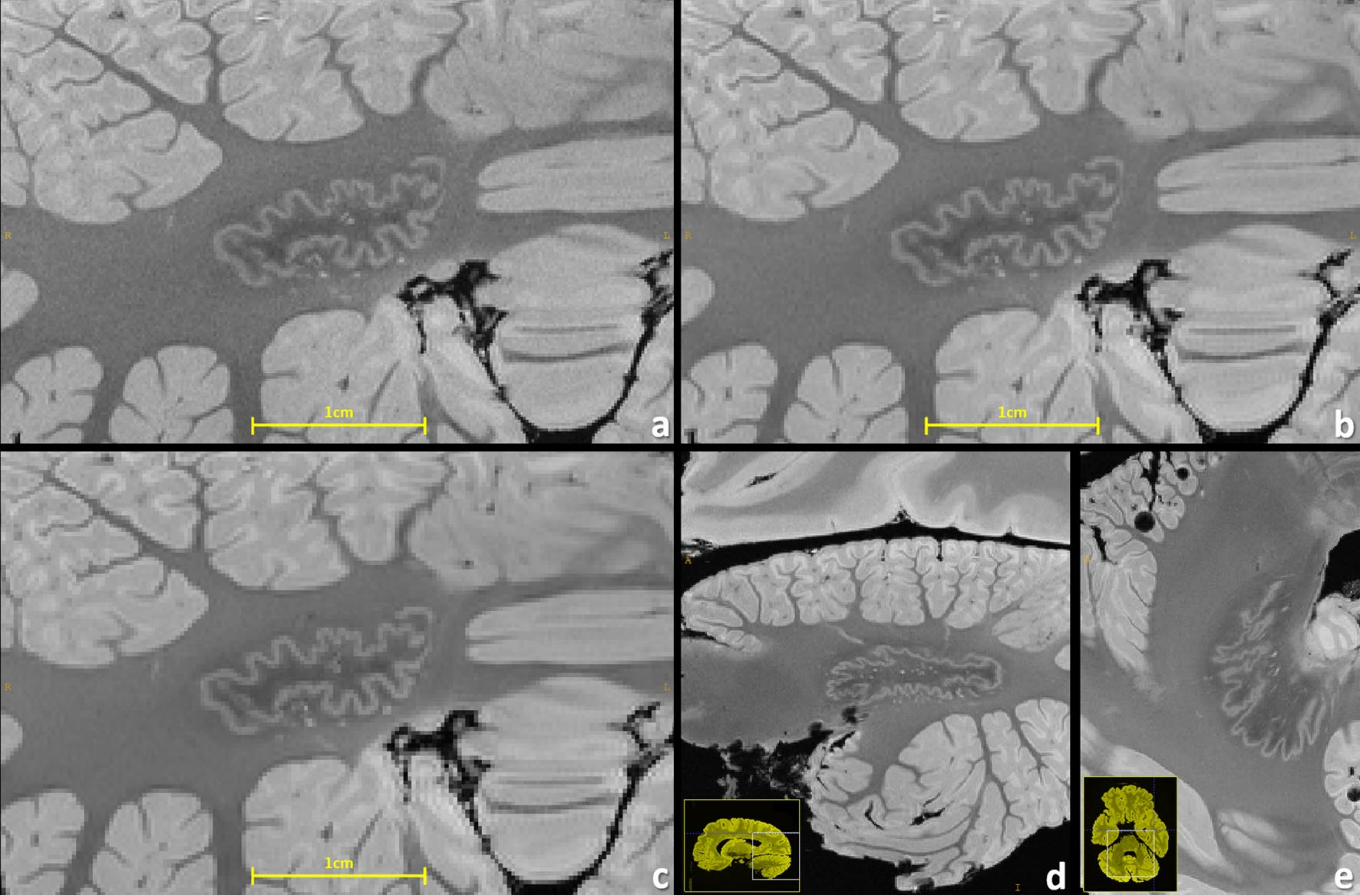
Brain #1, comparison of resolving power visualized at the cerebellar dentate nucleus with 160 µm (a), 200 µm (b), and 240 µm (c) resolution. Particularly for the 240 µm resolution the structures get pixelated, demonstrating that there is still gain in increasing even such ultra-high resolutions for brain MRI. A sagittal reformation (d) and a transverse slice (e) of the dentate nucleus are also depicted (200 µm resolution).
